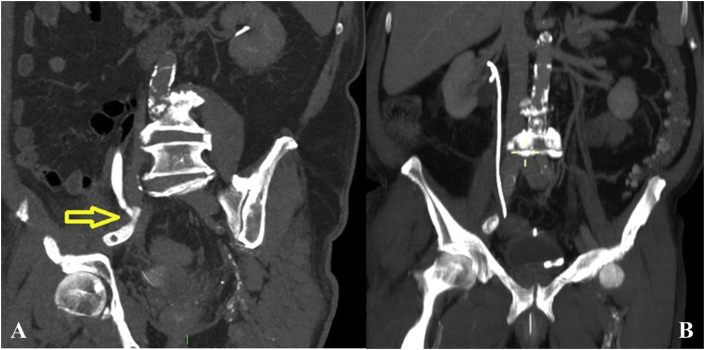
A: CT scan that evidence the distal right sided ureteral obstruction in correspondence of right external iliac artery. B: The double J ureteral stent positioned.
